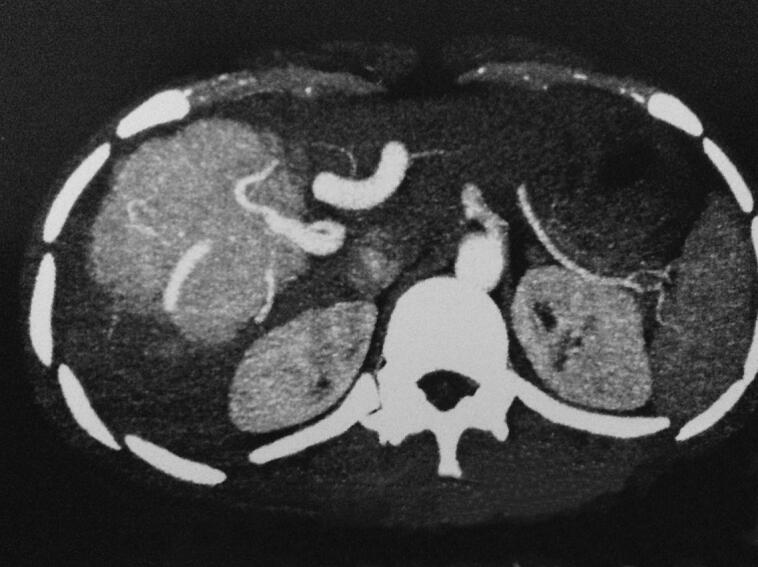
Follow-up CT-scan demonstrating significant reduction in lesion size post-arterial embolization.
